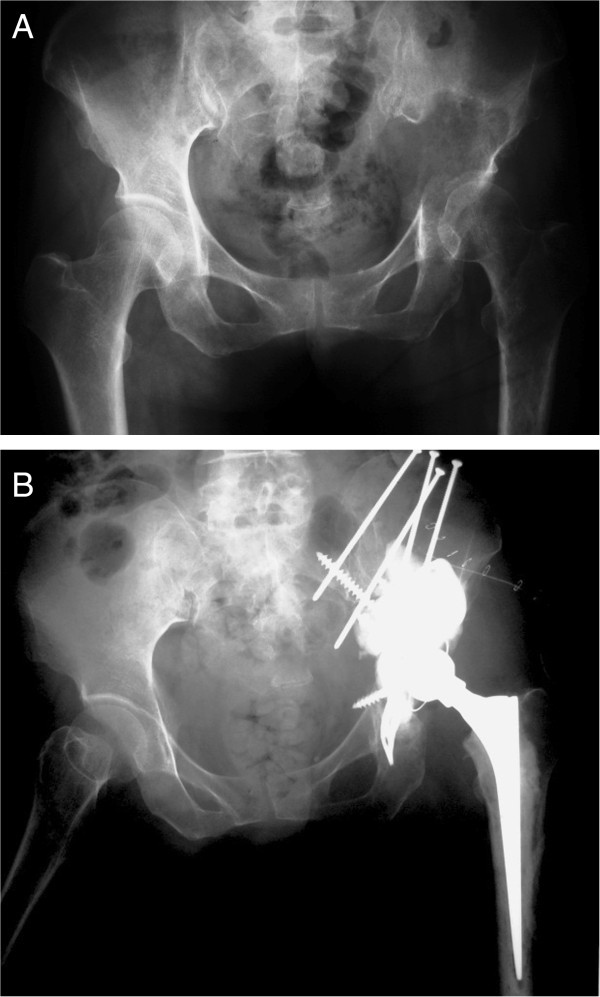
Radiograph of left hip of a 58 year-old man with class III periacetabular metastatic disease from hepatocellular carcinoma. (A) preoperative radiograph showed a large lesion involving the superior dome and medial wall. (B) after reconstruction with anti-protusio cage, screws and total hip arthroplasty. The patient was able to walk with a cane but died of disease at 12 months after the surgery without any complication.
